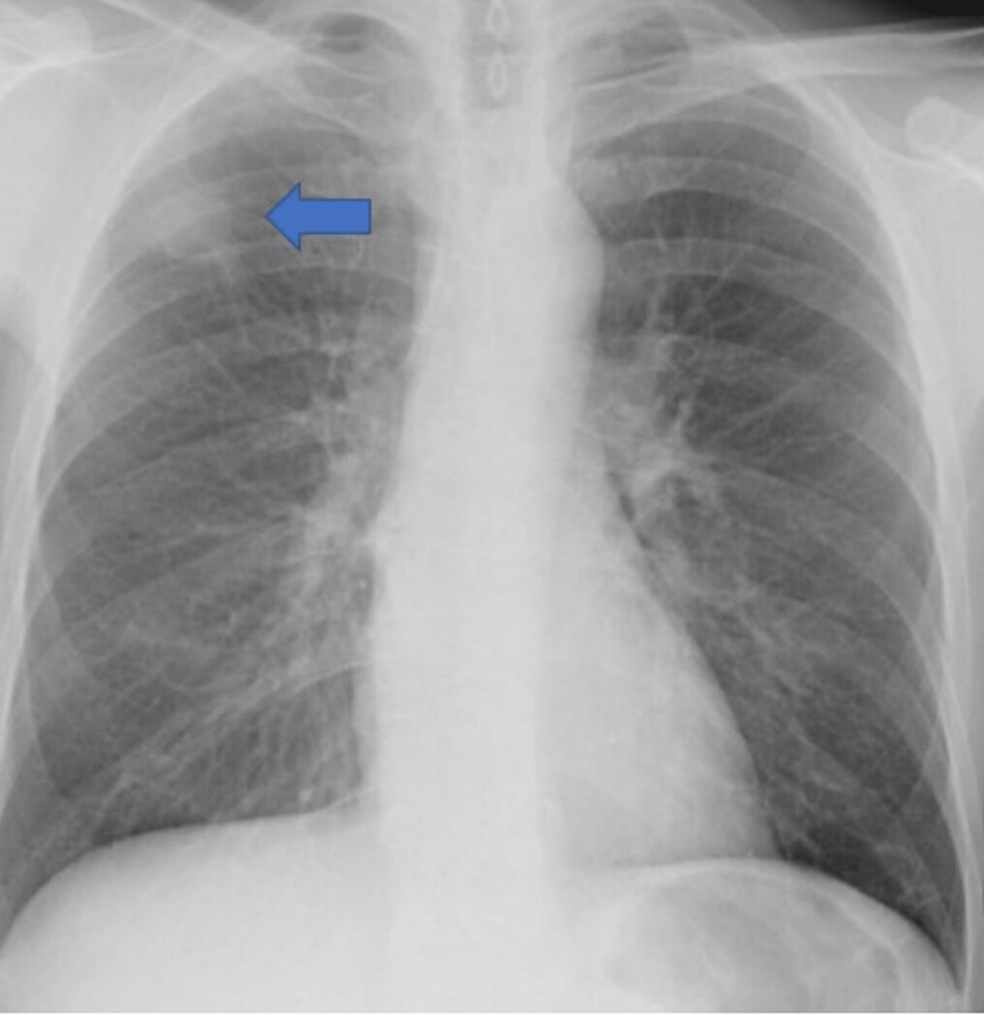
Plain X-ray of chest The image shows the pulmonary nodule in the right upper lung lobe (blue arrow).
